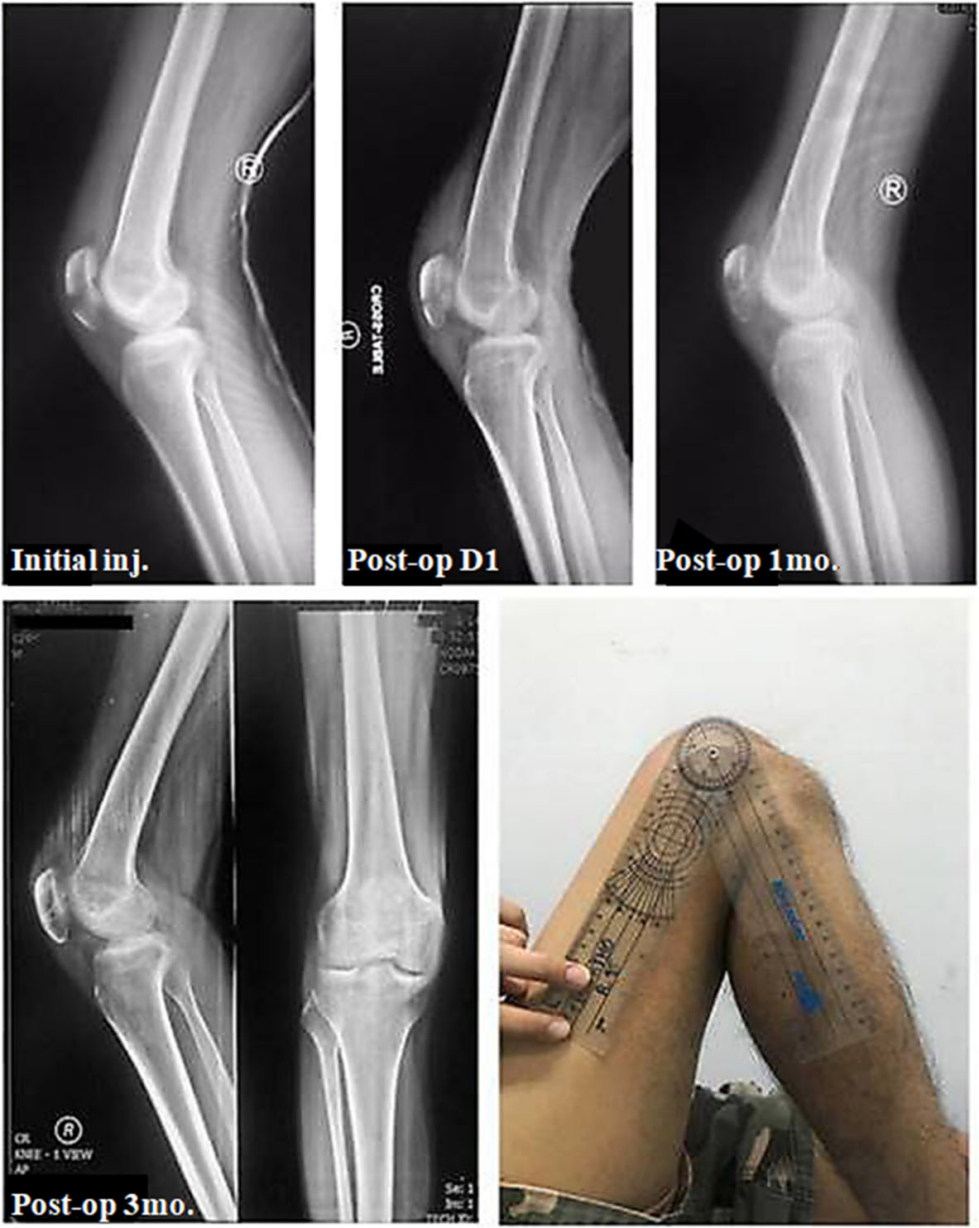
A 29-year-old male suffering from an indirect injury of the right patella. The initial radiograph shows a displaced transverse fracture of the patella. Postoperative radiographs reveal no intra-articular stepping and no displacement of the fracture site. The fracture healed within 3 months. Physical examination shows 0–130° of knee motion without pain. The ROM difference is −20°. Eventually, the patient gained full motion in 6 months
